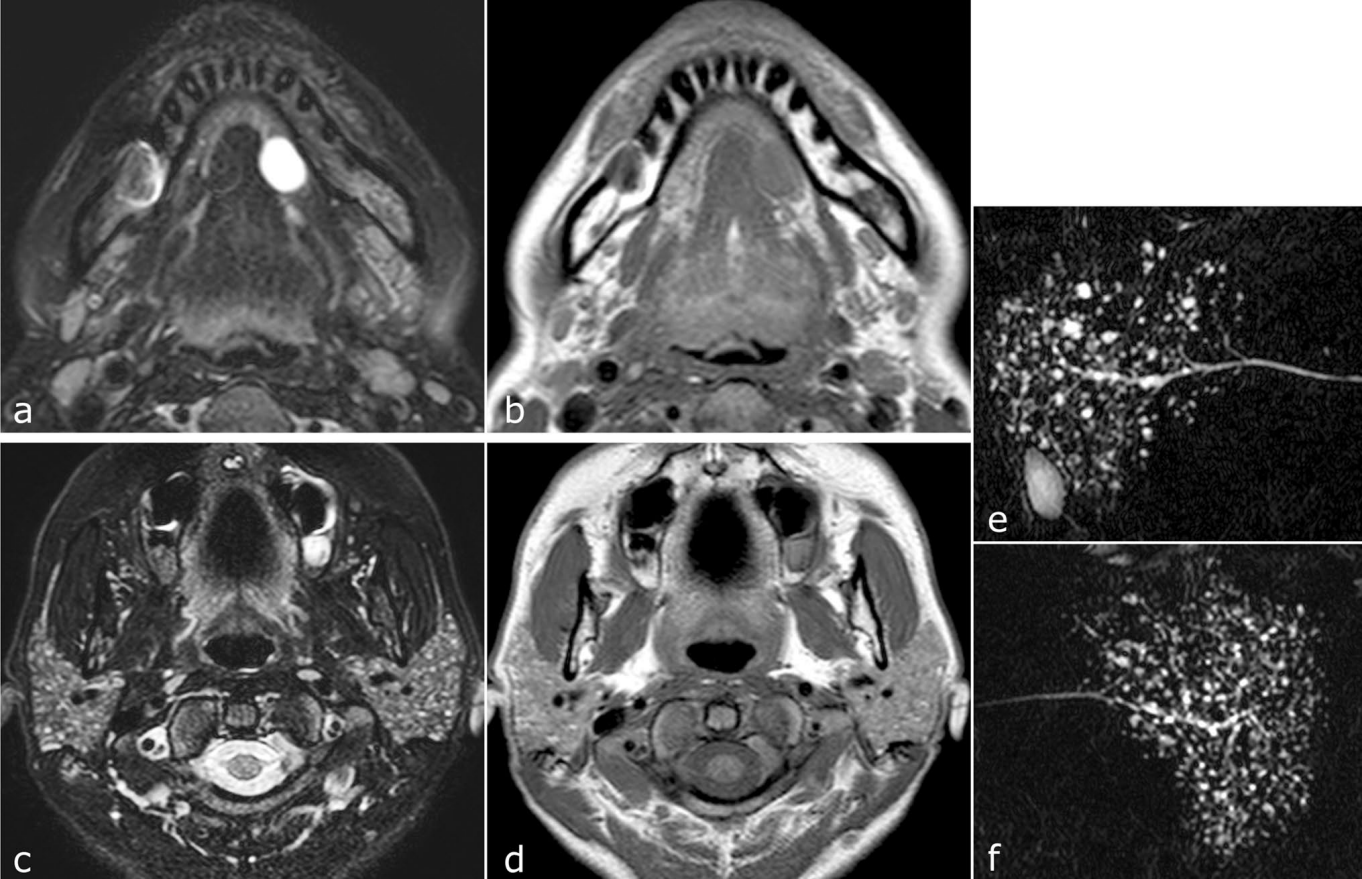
Magnetic resonance images of a 38 year-old woman with simple ranula who was not definitively diagnosed with SS but who had MRI findings strongly suggestive of SS (Case 5 in Table 1). a Axial fat-suppressed T2-weighted MR images show a homogeneous high-intensity, well-circumscribed cystic lesion in the left sublingual space. b Axial T1-weighted image shows bilateral atrophy of the submandibular glands resulting from severe fat degeneration. c Axial fat-suppressed T2-weighted image shows multiple very small high-intensity spots in parenchyma of the bilateral parotid glands. d Axial T1-weighted image shows slightly inhomogeneous parenchyma with fat deposition in the bilateral parotid glands e, f MR sialography shows punctate sialoectasia in right (e) and left (f) parotid glands.
